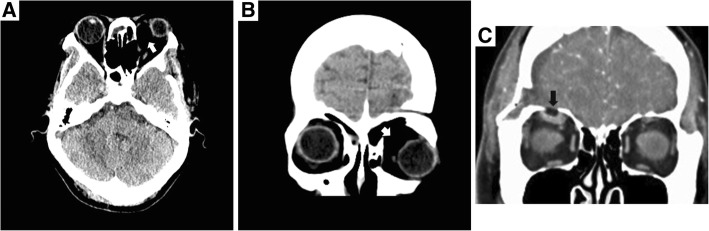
CT findings of bone wax in orbit in our case (4-a,4-b white arrows) is consistent with hypodense or fat density lesions as described in previous paper [5] (4-c black arrow)
